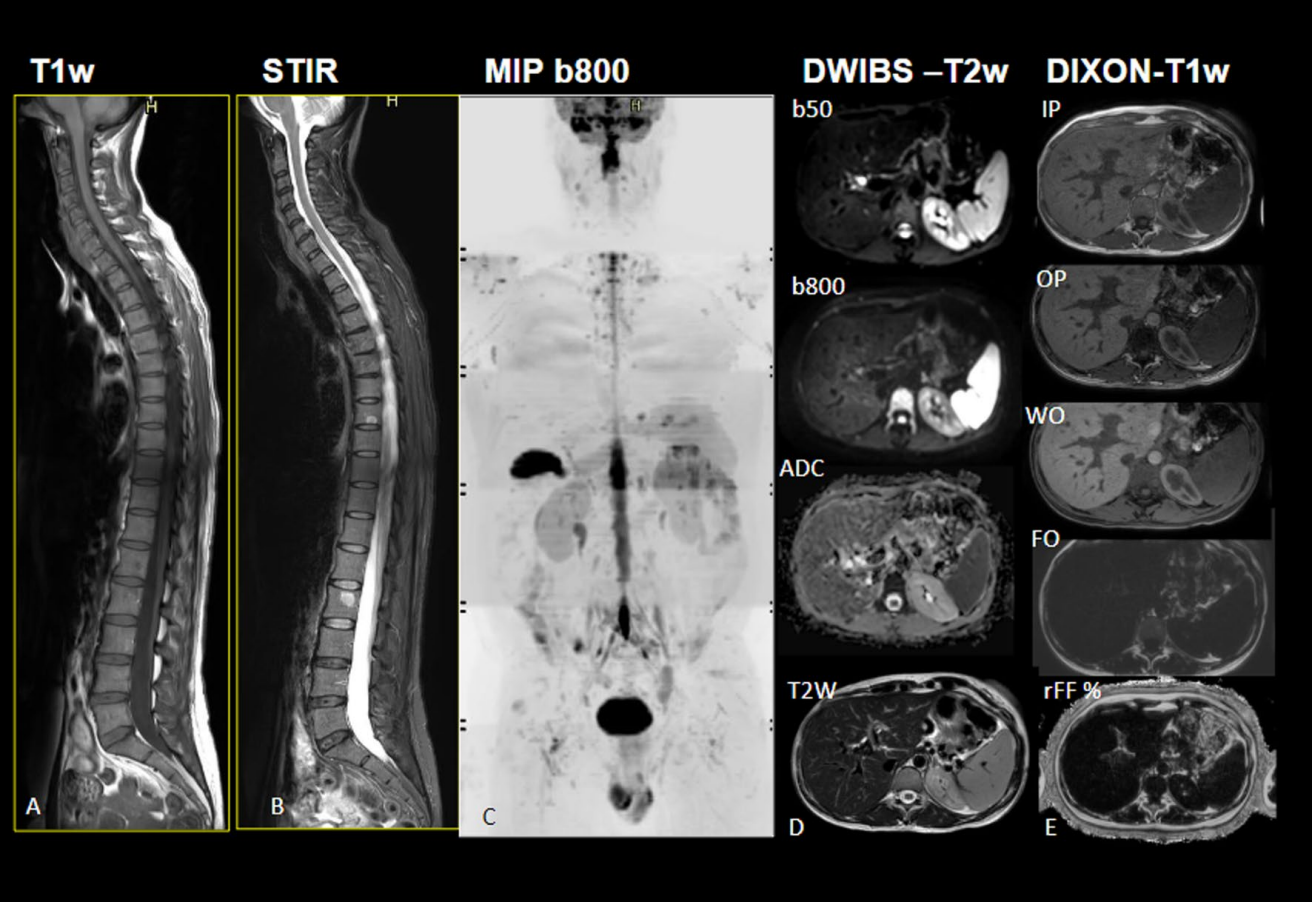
Images were acquired according to our WB-MRI protocols
